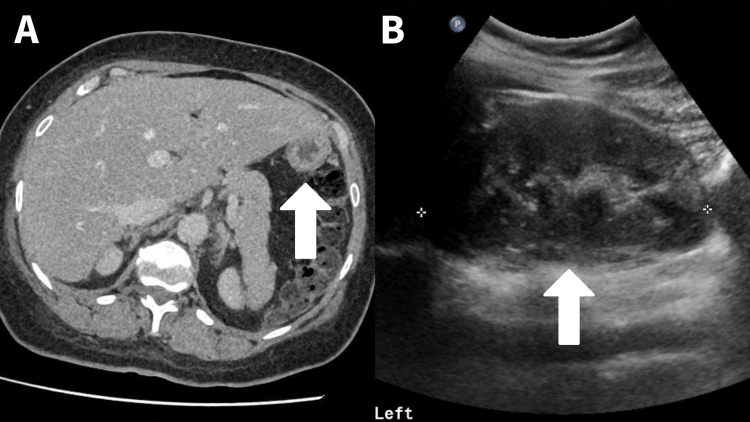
Evolution of splenic findings on imaging. (A) CT abdomen (2025) showing absence of splenic tissue. The arrow indicates the expected splenic bed/left upper quadrant, highlighting the absence of splenic tissue (some redundant tissue visible). (B) Ultrasound image (2011) demonstrating a normal spleen. The arrow indicates the normal spleen, confirming that splenic anatomy was previously preserved. CT, computed tomography
